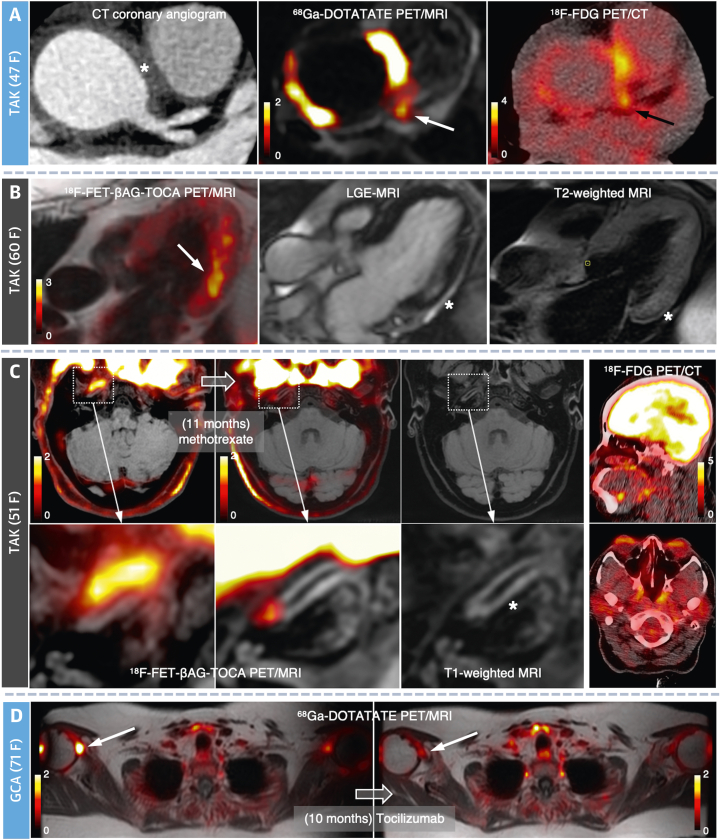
Varied Patterns of LVV Involvement SST_2_ PET/MRI signals (white arrows) in (A) the aortic root and left main coronary artery with adjacent periaortic thickening (asterisk) and ^18^F-FDG PET uptake (black arrow) in a patient with active TAK, (B) the basal inferolateral left ventricular myocardium in a patient with inactive TAK and subclinical myocarditis confirmed by mid-wall late gadolinium enhancement (asterisk) and increased T_2_-edema signal (asterisk), (C) the thickened intracranial portion of the right internal carotid artery (asterisk) in a patient with TAK and previous stroke in whom ^18^F-FDG failed to detect this abnormality, and (D) the right glenohumeral joint in a patient with GCA and polymyalgia rheumatica symptoms. Note that there is a reduction in PET signal intensity following immunosuppressive treatment in C and D. Image scale bars indicate SUV. LGE = late gadolinium enhancement; other abbreviations as in Figures 1 and 2.
